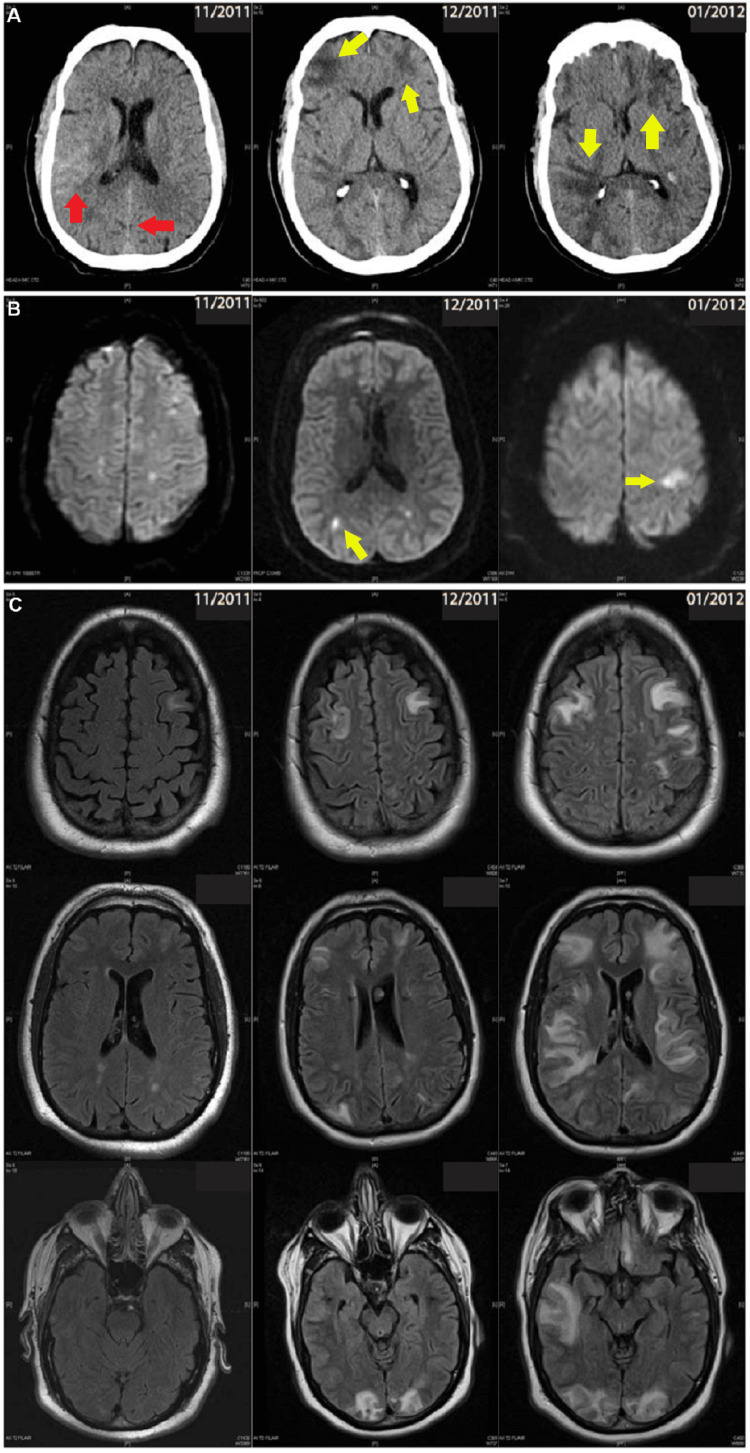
Dynamic progression of different types of brain lesions in a patient who did not receive chemotherapy (case 4). (A) SAH (red arrow), IPH (red arrow), and cerebral edema (yellow arrow) in the patient with a different clinical stage, (B) multi-foci of restrict diffusion representing acute ischemic infarct (light green arrow), (C) progression of T2/FLAIR hyperintensity lesions in a different clinical stage. SAH - Subarachnoid Hemorrhage; IPH - Intraparenchymal Hemorrhage
